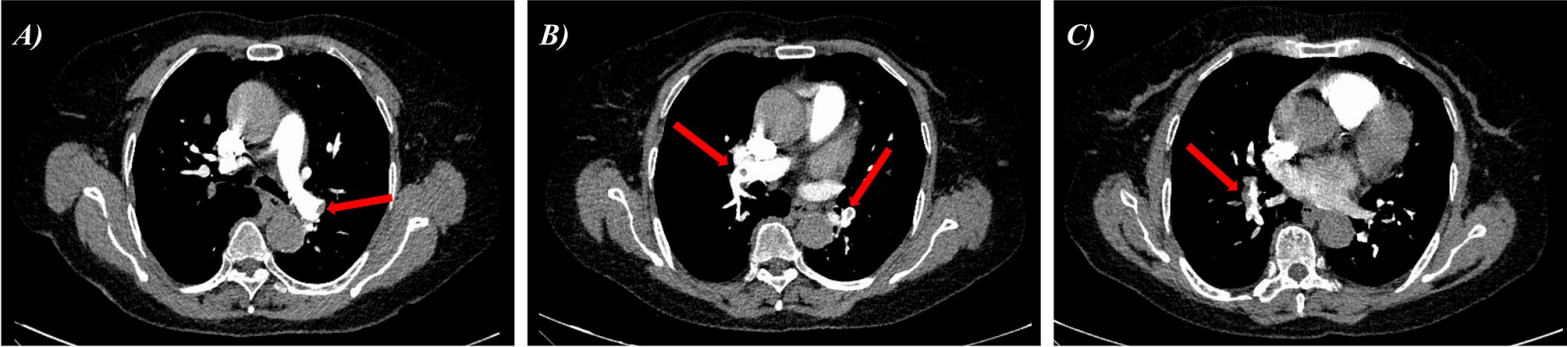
CTPA images showing pulmonary artery thrombi. The red arrows in Panels A, B, and C indicate thrombi at various levels extending to the distal end of the pulmonary artery. CTPA = computed tomography pulmonary angiography
